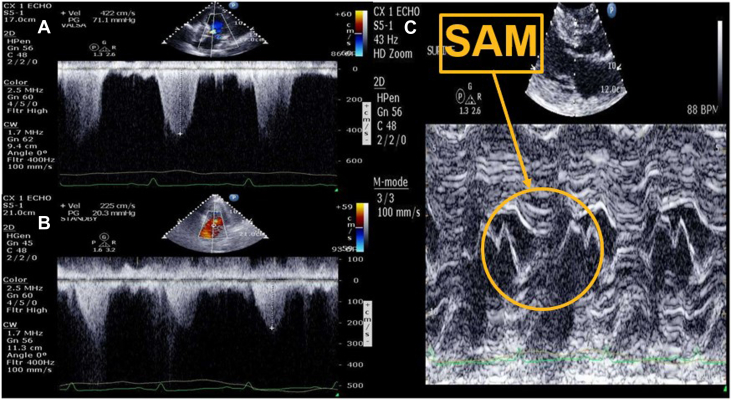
Transthoracic Echocardiogram With IABP at 1:2 Augmentation, Vasopressors Weaned Off, and IVF Administration (A) Continuous-wave Doppler showing LVOTO. (B) Continuous-wave Doppler showing improvement of LVOTO while IABP on standby. (C) M-mode showing SAM. IABP = intra-aortic balloon pump; IVF = intravenous fluids; LVOTO = left ventricular outflow tract obstruction; SAM = systolic anterior motion.
